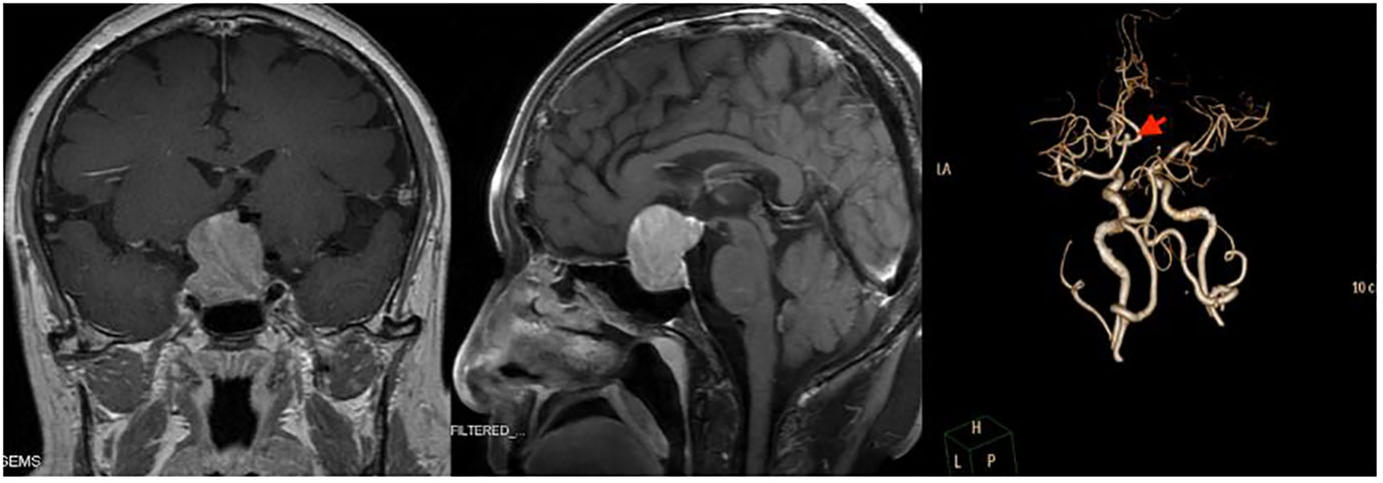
Preoperative imaging examination of the reported patient. Left and Middle: Sagittal and coronal T1 MR imaging after injection of gadolinium. Both pictures showed massive masses in the sellar fossa and suprasellar cistern. Right: Computed Tomography Angiography (CTA) showed an aneurysm at the anterior edge of the anterior communicating artery, with a diameter of about 0.5cm.
